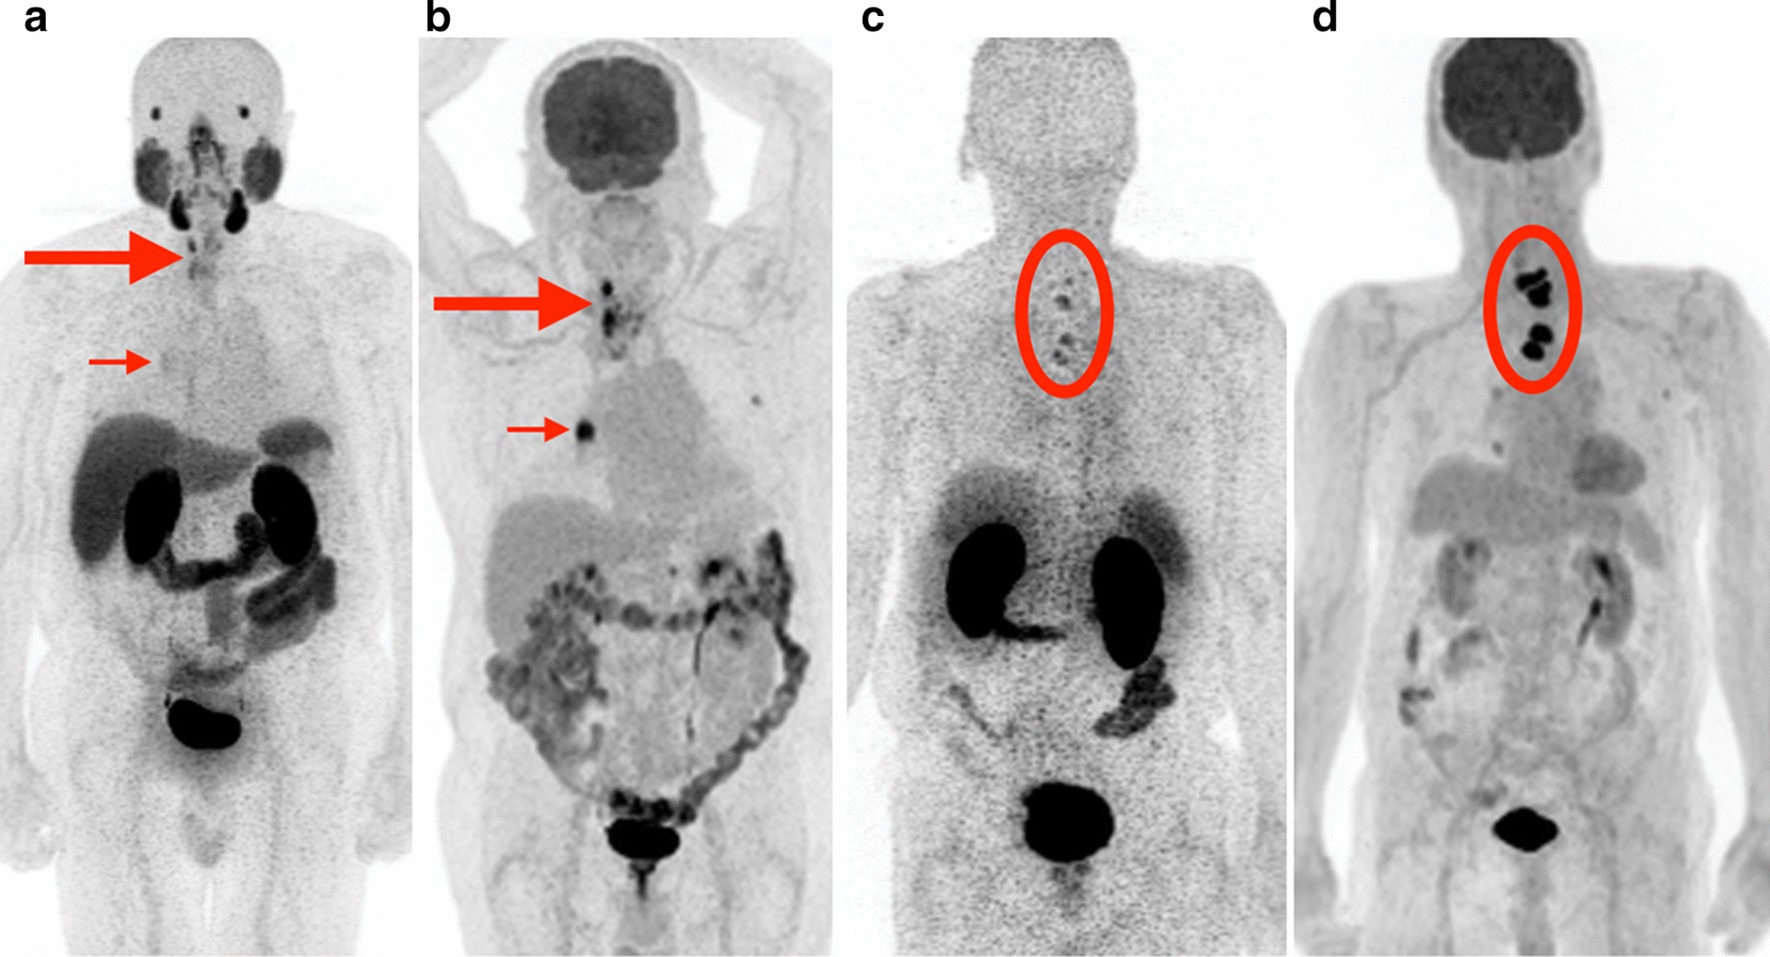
Patients with metastatic thyroid cancer with greater FDG uptake than PSMA uptake: examples. a, b [^68^Ga]Ga-PSMA-11 PET MIP (a) and 2-[^18^F]FDG PET MIP (b) in a patient (Patient 4) with anaplastic thyroid carcinoma. Thyroid bed recurrence (large arrows) and right hilar nodal metastasis (small arrows) are more avid on FDG (median SUVmax 14.3) than PSMA (median SUVmax 3.3). c, d [^68^Ga]Ga-PSMA-11 PET MIP (c) and 2-[^18^F]FDG PET MIP (d) in a patient (Patient 1) with papillary thyroid carcinoma. Multiple lower cervical and upper thoracic vertebral body metastases (circled) are more avid on FDG (median SUVmax 21.1) than on PSMA (median SUVmax 9.0)
